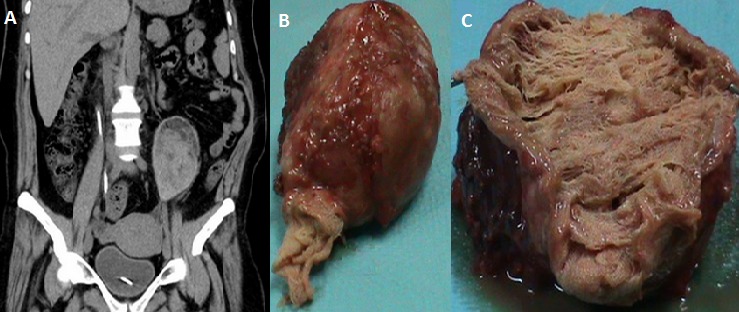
Gossypiboma or retained surgical sponges: A) unenhanced abdominal computed tomography showing a well-encapsulated ovoid heterogeneous mass, located within the left lumbar region; B) resected mass; C) pathological examination showing retained surgical sponges surrounded by fibrous capsule
